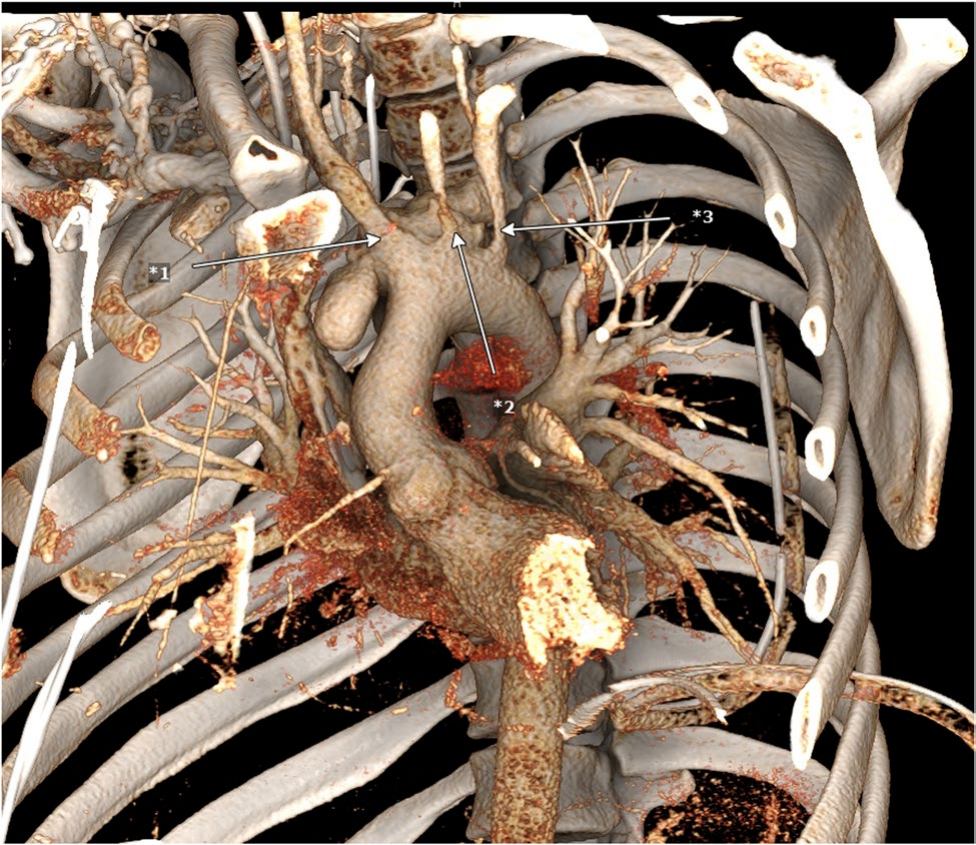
3D reconstruction of the CT scan, *1 brachiocephalic trunk; *2 left common carotid artery, *3 left subclavian artery
